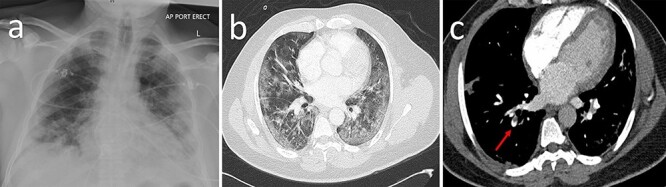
Pulmonary disease in COVID-19. (a) Chest X-ray demonstrating severe bilateral patchy infiltrates. (b) Extensive subpleural and peribronchovascular ground-glass infiltrates on computed tomography. (c) Filling defect in the right lower pulmonary subsegmental artery on CT pulmonary angiography (red arrow).
